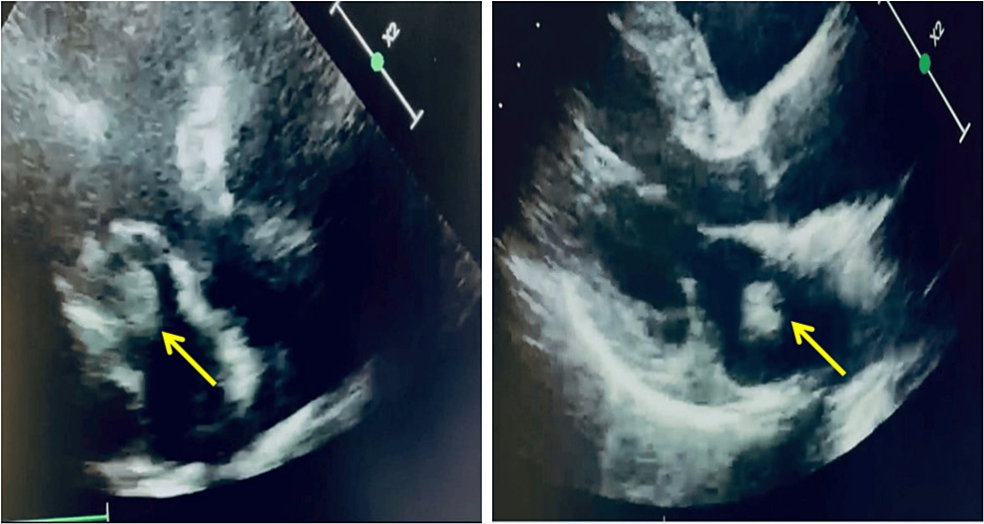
Transthoracic echocardiography of the cardiac mass pre-surgical intervention Arrow: the cardiac mass.
